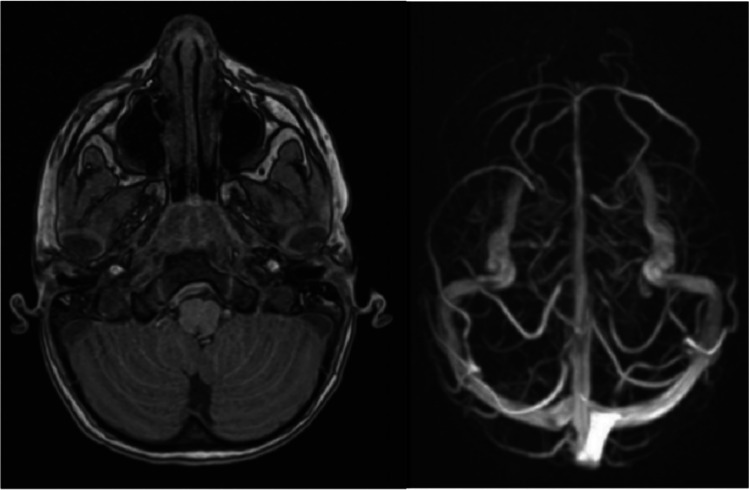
Interval MRI at 16 months confirmed complete resorption of the EDH and resolution of the CVST.
